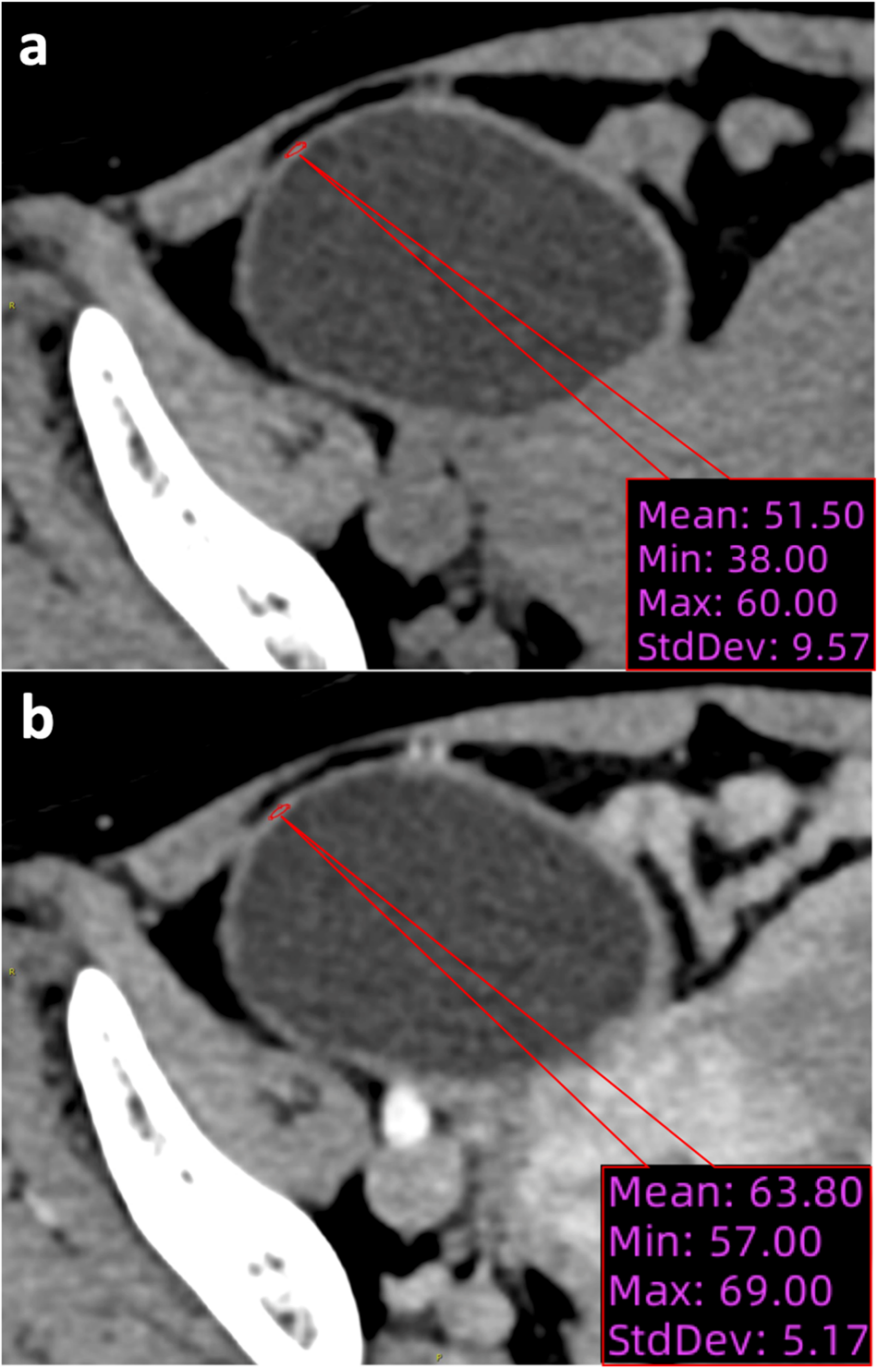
Attenuation measurement in contrast-enhanced CT of a patient (age: 53 years) with a chief complaint of abdominal pain for 1 day. Surgically and pathologically confirmed twisted ovarian cyst. a Non-contrast phase (attenuation: 51.5 HU). b Portal-venous phase (attenuation: 63.8 HU). The ΔHU_PV-NC_ is 12.3 HU
